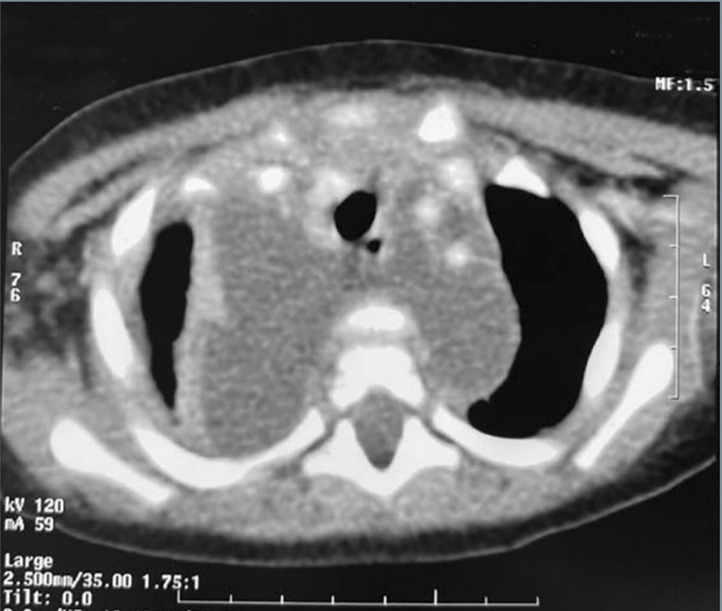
a thoracic CT scan showing a collected diffuse mediastinitis spread to the cervical region associated with a right pleural empyema and pulmonary condensation
